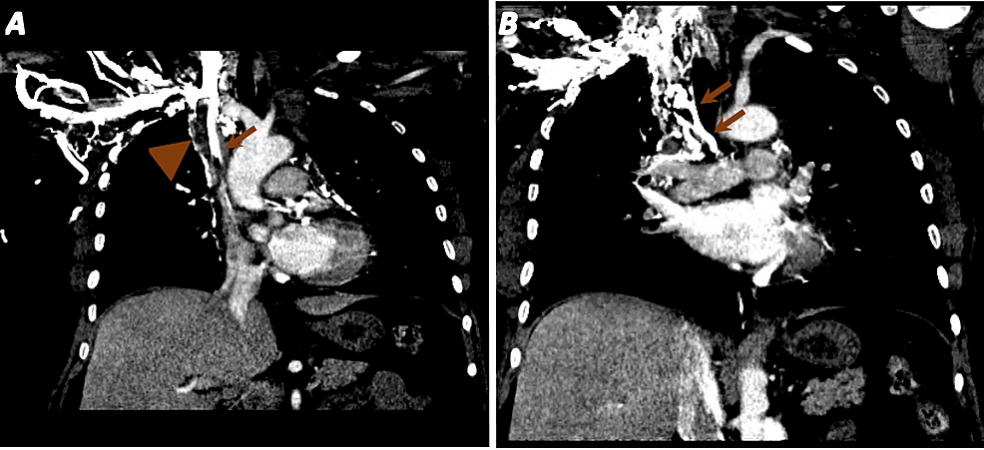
CT chest with contrast showing a filling defect in the superior vena cava (arrowhead) and a right-sided infusion catheter port with the tip at the cavoatrial junction (arrow) (A). Extensive chest wall and mediastinal collaterals (arrows) (B) CT: computed tomography
